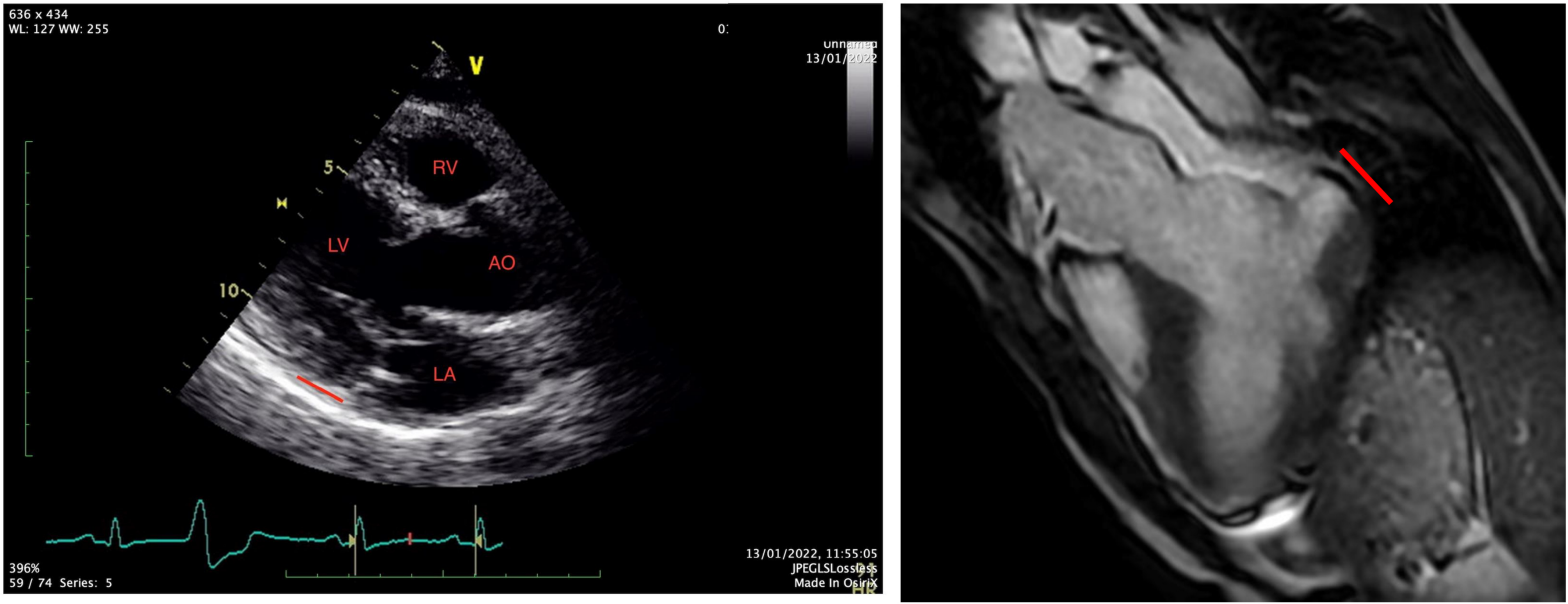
TTE and MRI, MAD noted with the red line.
